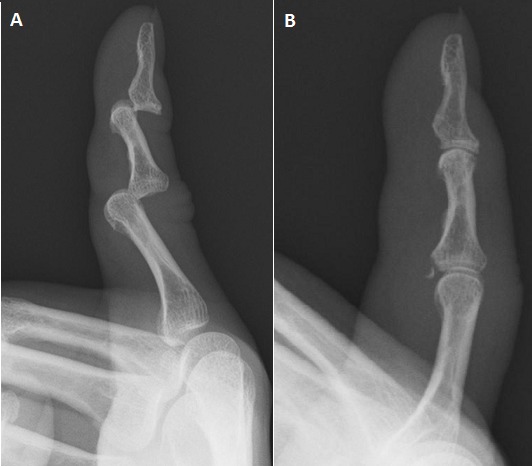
Case 1 (A) X-rays of the little finger showing dorsal dislocation of both the proximal and distal interphalangeal joints (step-ladder deformity); (B) Post-reduction X-rays confirming that both joints are well reduced, but the PIP joint had a volar rim fracture
